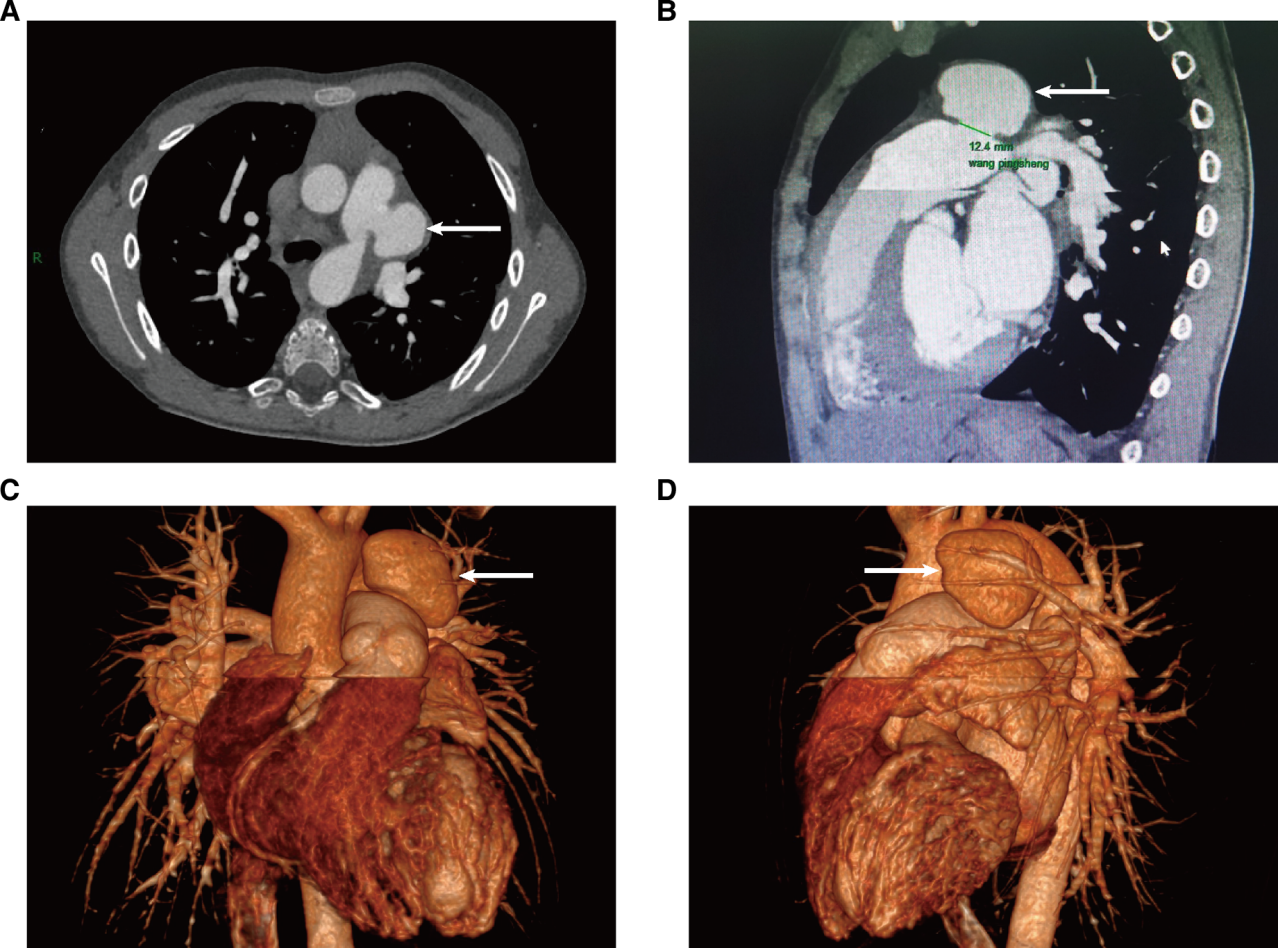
Cardiac contrast-enhanced CT on 14 November 2022; the PAA is indicated by the arrow. (A) Cross-section of CT images; (B) sagittal plane of a CT image; (C,D) 3D reconstruction of a CT image.
